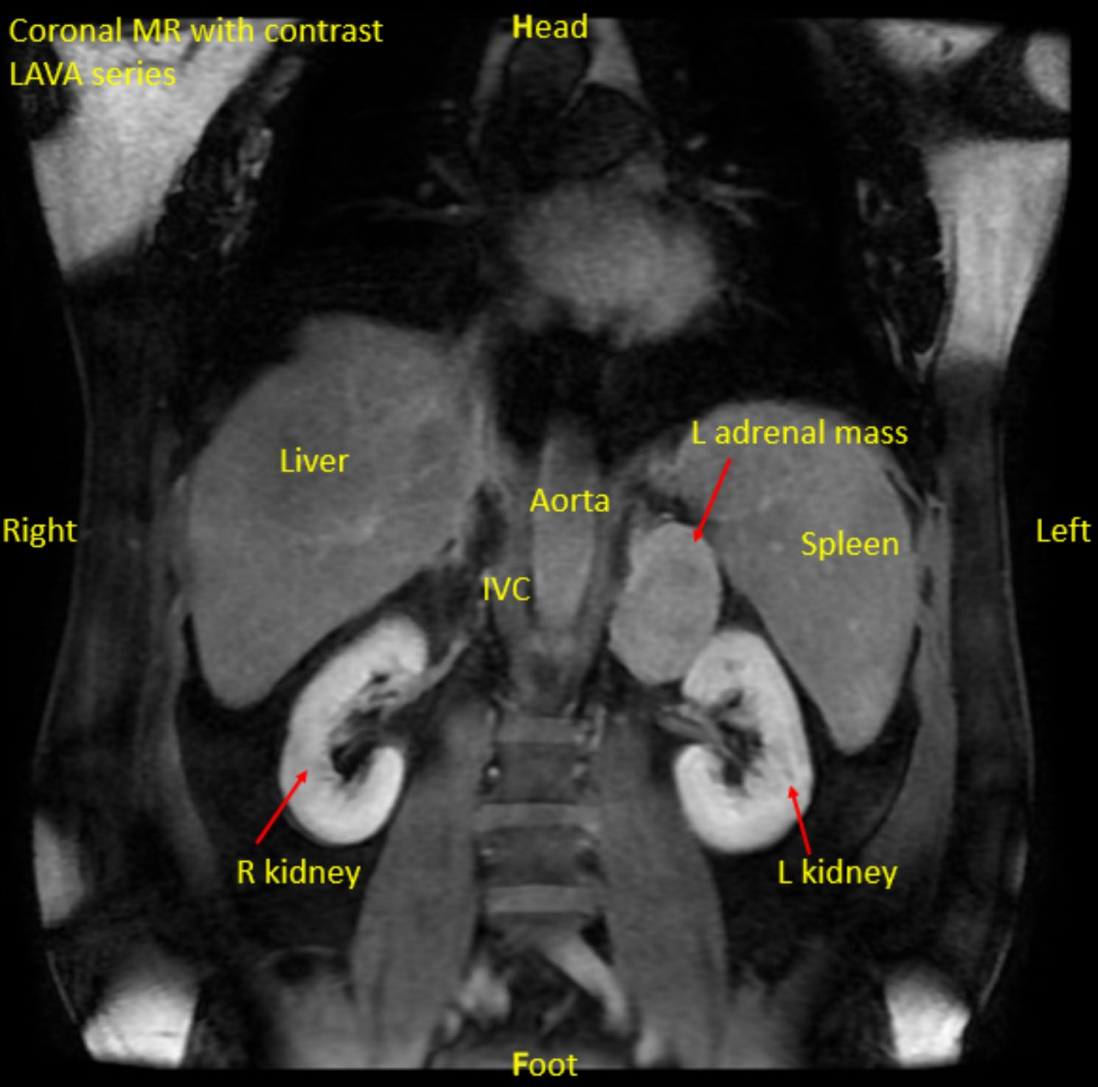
MRI abdomen with contrast, coronal, labeled
